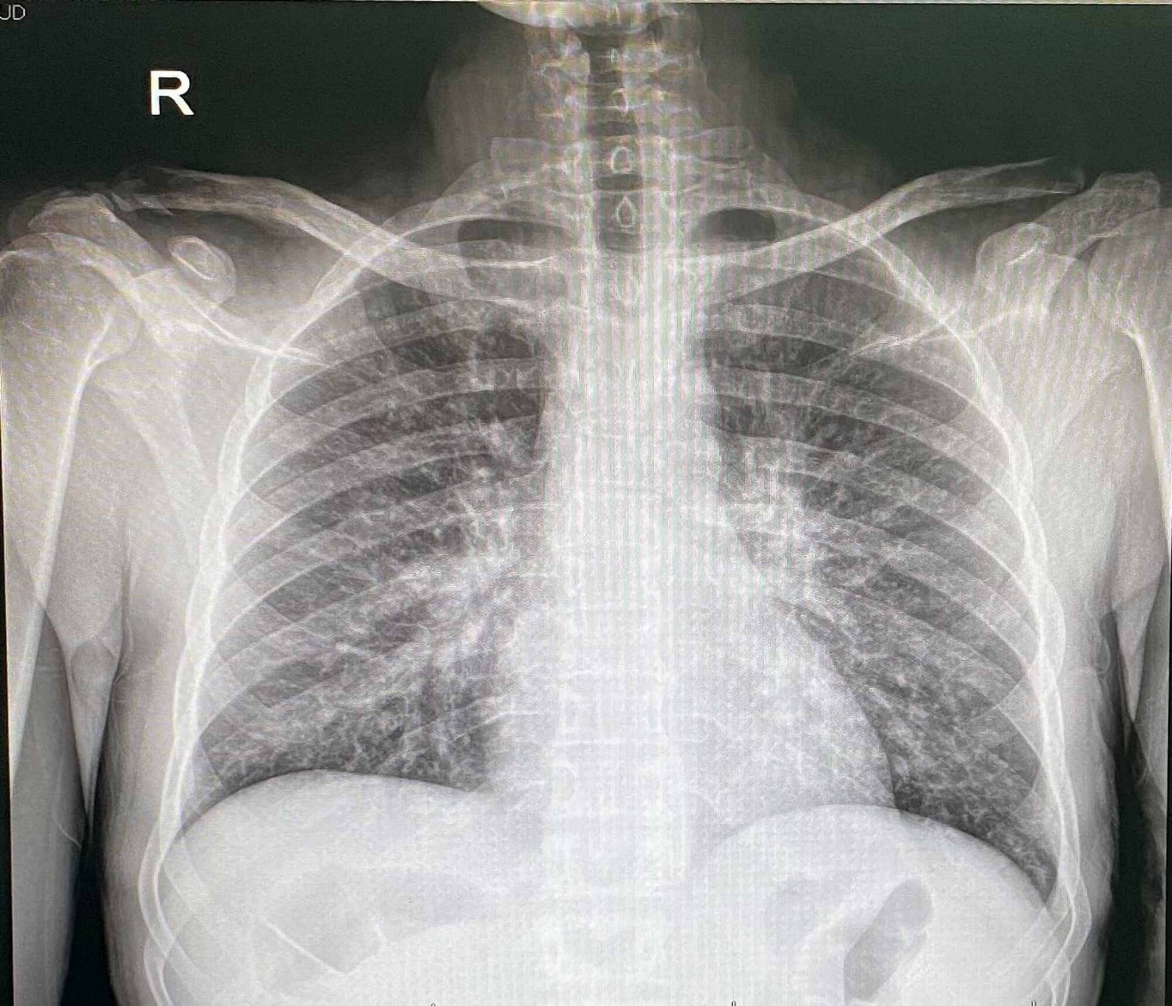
Posteroanterior chest radiograph showing bilateral reticulonodular infiltration
